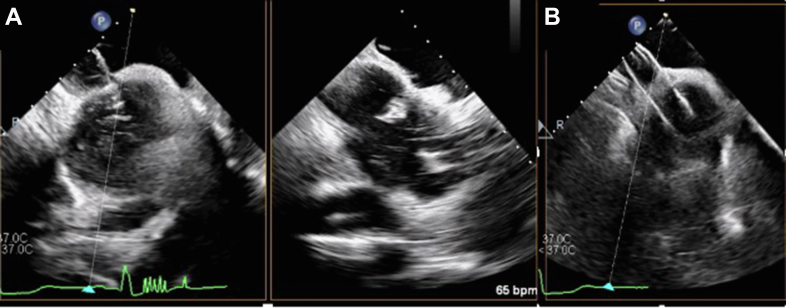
Trans-septal Puncture Intracardiac echocardiography showing the trans-septal puncture (A) followed by atrial septostomy (B).
